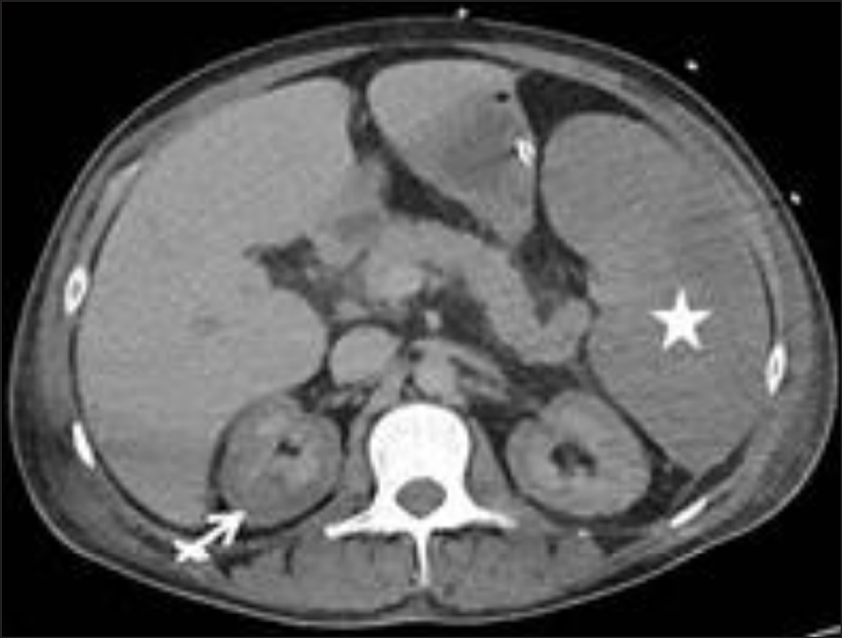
Axial contrast-enhanced CT in the venous phase showing the same renal enhancement pattern as in the arterial phase with a non enhancing renal cortex (white arrow). Also note splenomegaly (asterisk).
